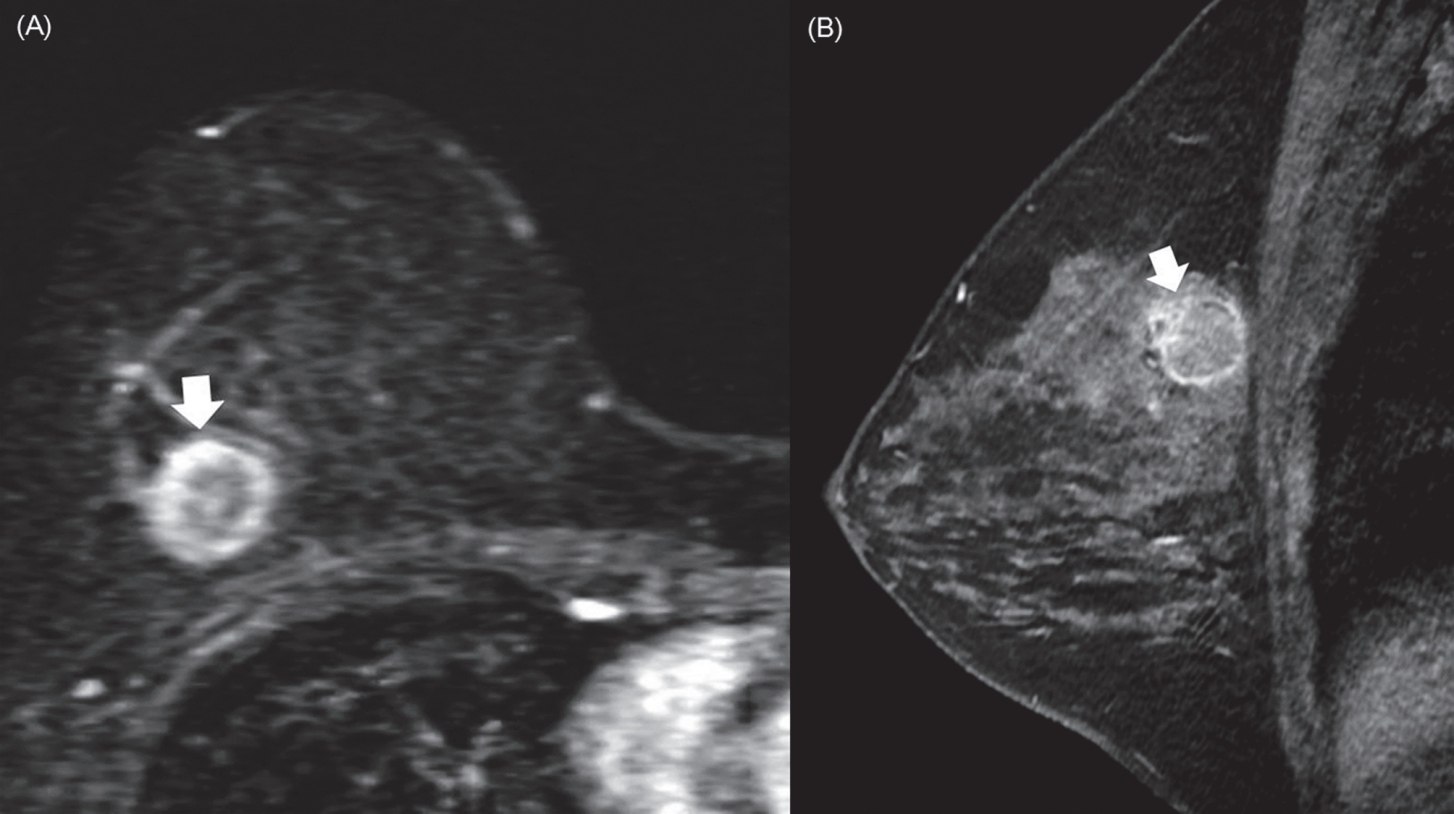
A triple-negative breast cancer in the posterior location. A triple-negative breast cancer in the posterior location of the right breast in a 41-year-old woman. (A) Sagittal early contrast-enhanced nonsubtracted T1-weighted MR image with fat suppression shows a 1.5 cm round mass (arrow) with rim enhancement. Note the tumor location in the immediate prepectoral region. (B) Mass (arrow) is also seen on subtracted axial reformatted image.
